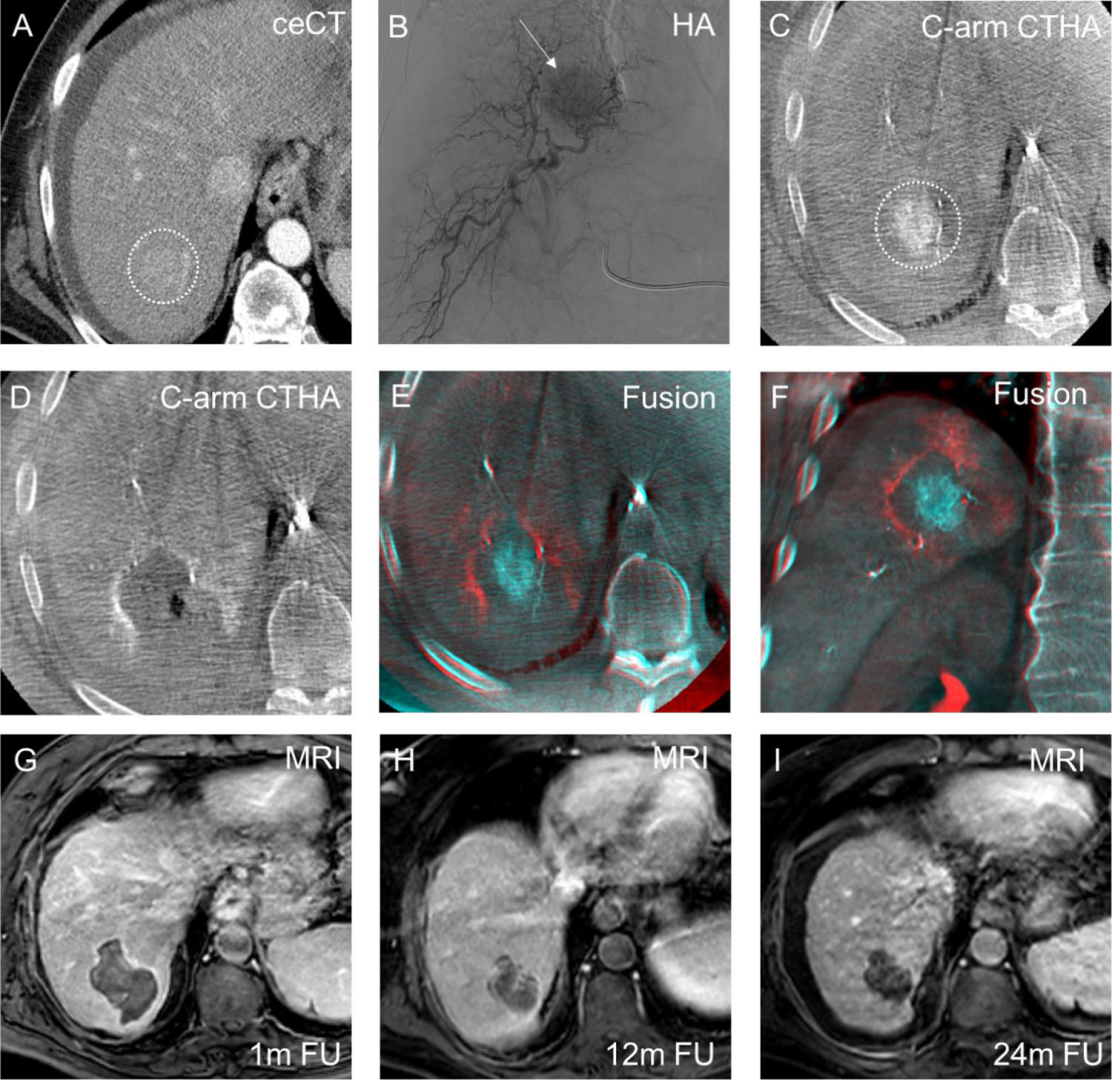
Example of a HepACAGA procedure for a HCC with a tumor size of 36 mm located in segment VII/VIII. A The lesion is moderately visible on contrast-enhanced CT (ceCT) during the arterial phase (white circle) 1 month prior to the procedure B The lesion is visualized with hepatic arteriography (HA) (white arrow) during the procedure C Intraprocedural C-arm CT hepatic arteriography (C-arm CTHA) provides adequate visualization of the target lesion (white circle) D The ablation zone after 15 min ablation at 150W is depicted with C-arm-CTHA immediately after ablation E,F Fusion of pre- and post-ablation C-arm CTHAs using XperGuide software in the axial and coronal planes confirms adequate ablation margins G,H,I Follow-up (FU) MRI (T1 with gadolinium) at 1 month, 12 months, and 24 months demonstrates no local tumor progression and a progressively shrinking ablation zone
